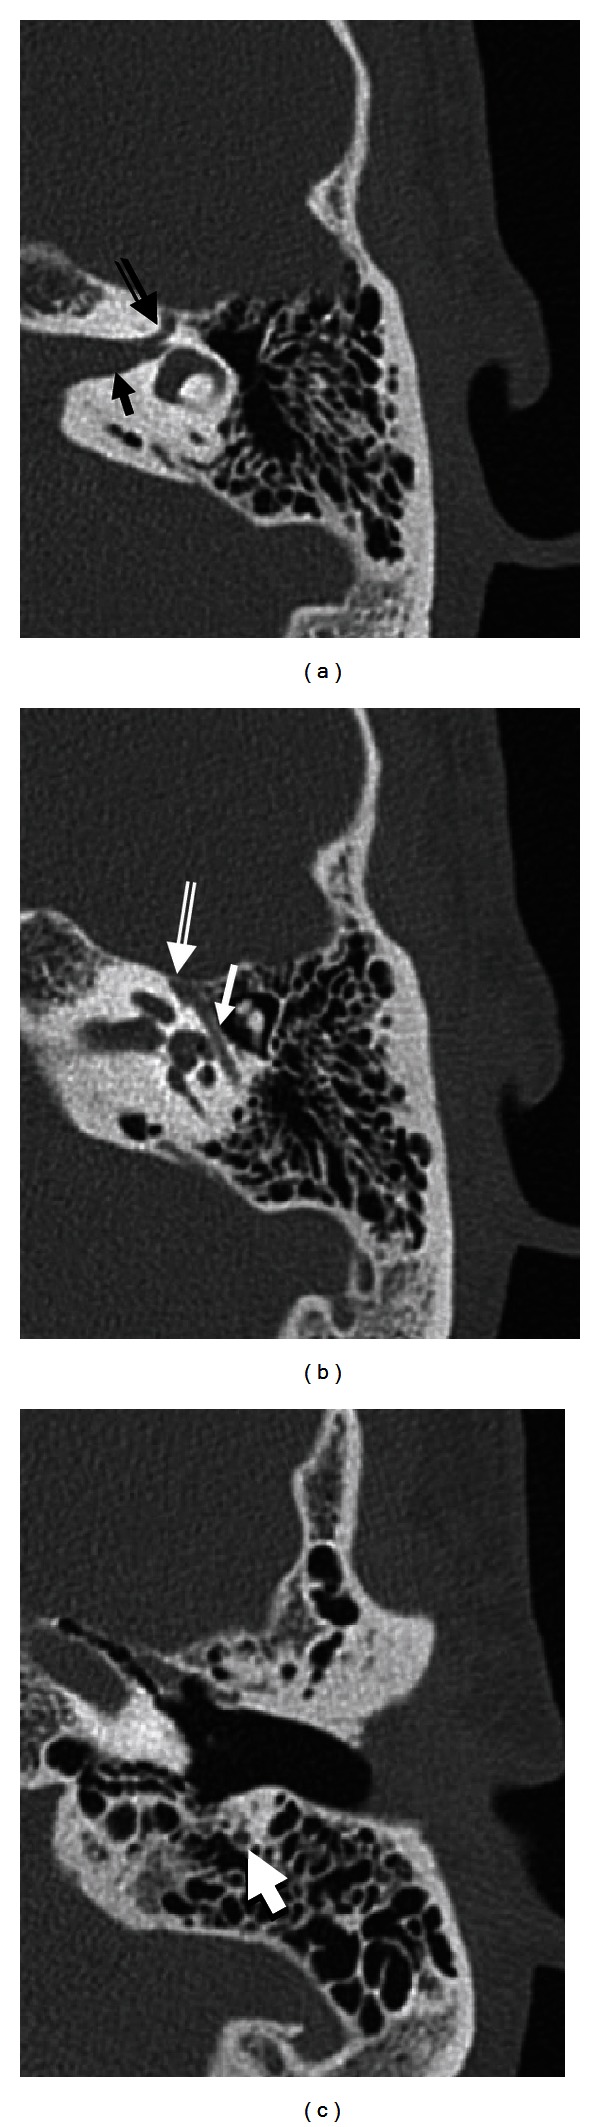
Normal facial nerve canal on CT. Axial temporal bone CT images demonstrate the intracanalicular (solid arrow in (a)), labyrinthine (double lined arrow in (a)), geniculate ganglion (double lined arrow in (b)), tympanic (solid arrow in (b)), and mastoid (arrow in (c)) segments of the facial nerve.
